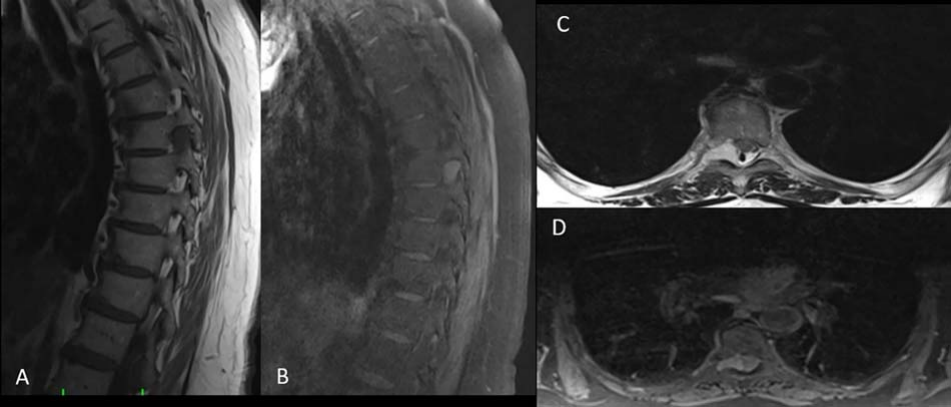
On preoperative MRI, the mass was (A) hypointense on T1-weighted sagittal images, (B) hyperintense on contrast-enhanced T2-weighted sagittal images, (C) hyperintense on T2-weighted axial images and (D) isointense on T1-weighted axial images.
